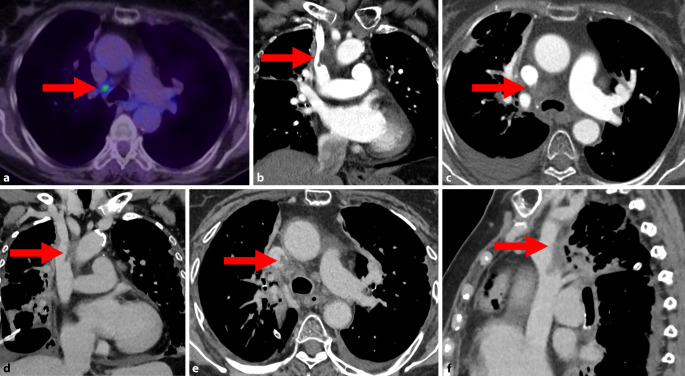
a Vitaler/hypermetaboler mediastinaler Lymphknoten (Station: 4R, *Pfeil*) in der PET-CT. Dies ist der Lymphknoten, der im Anschluss EBUS-gestützt punktiert wurde. b Erstdiagnose eines Hämatoms (*Pfeil*) nach Lymphknotenbiopsie in der CT des Thorax im koronaren Schnitt. c–f Verlaufs-CT mit Superinfektion des Hämatoms (c, *Pfeil*). Zudem flottierender Thrombus (d, *Pfeil*) in der VCS über der Punktionsstelle im koronaren Schnitt. Darstellung des Thrombus (axial und sagittal, *Pfeile* e, f). Zu sehen ist nebenbefundlich ein Pleuraerguss rechts. Im Verlauf wurde eine Pleuradrainage eingelegt, der Erguss ist entsprechend regredient
